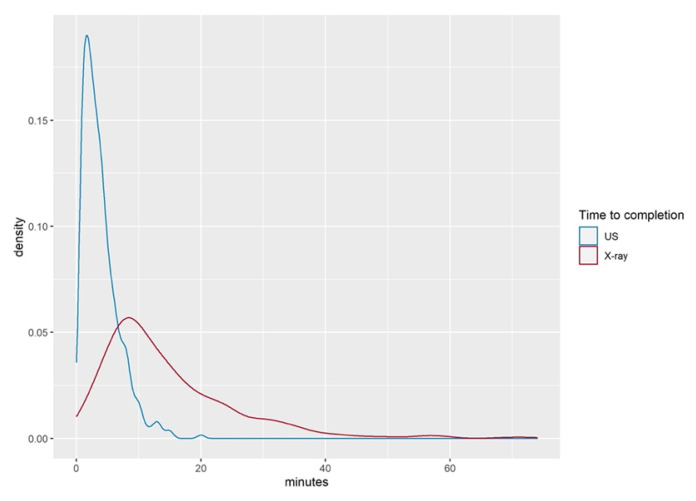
Time difference between ultrasound investigation and radiographic image study.
